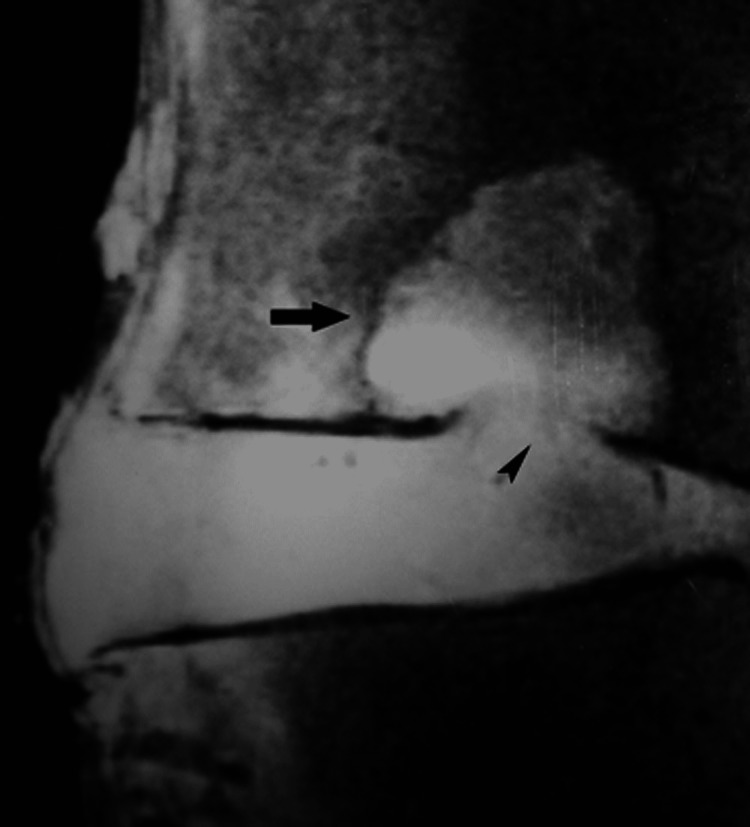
Schmorl nodes. Sagittal MR image demonstrates large cartilaginous (Schmorl) node (arrow). Note disruption of the midportion of the vertebral end-plate (arrowhead).
